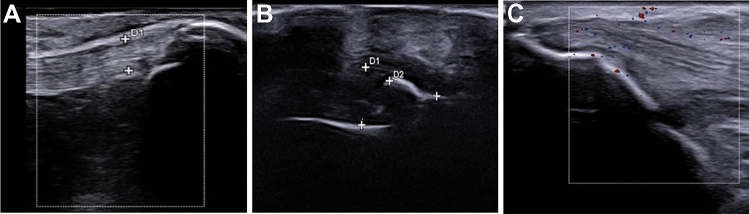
HFUS enthesis evaluation. A Thickened distal rotuleus tendon (d1: 4.5 mm) with irregular cortical profile without significant increase in the vascular pattern; B Thickened Achilles tendon (d1: 5.6 mm) with large calcification (d2; MASEI score = 3); C Normal fibrillar pattern of triceps tendon without significant Doppler signal
